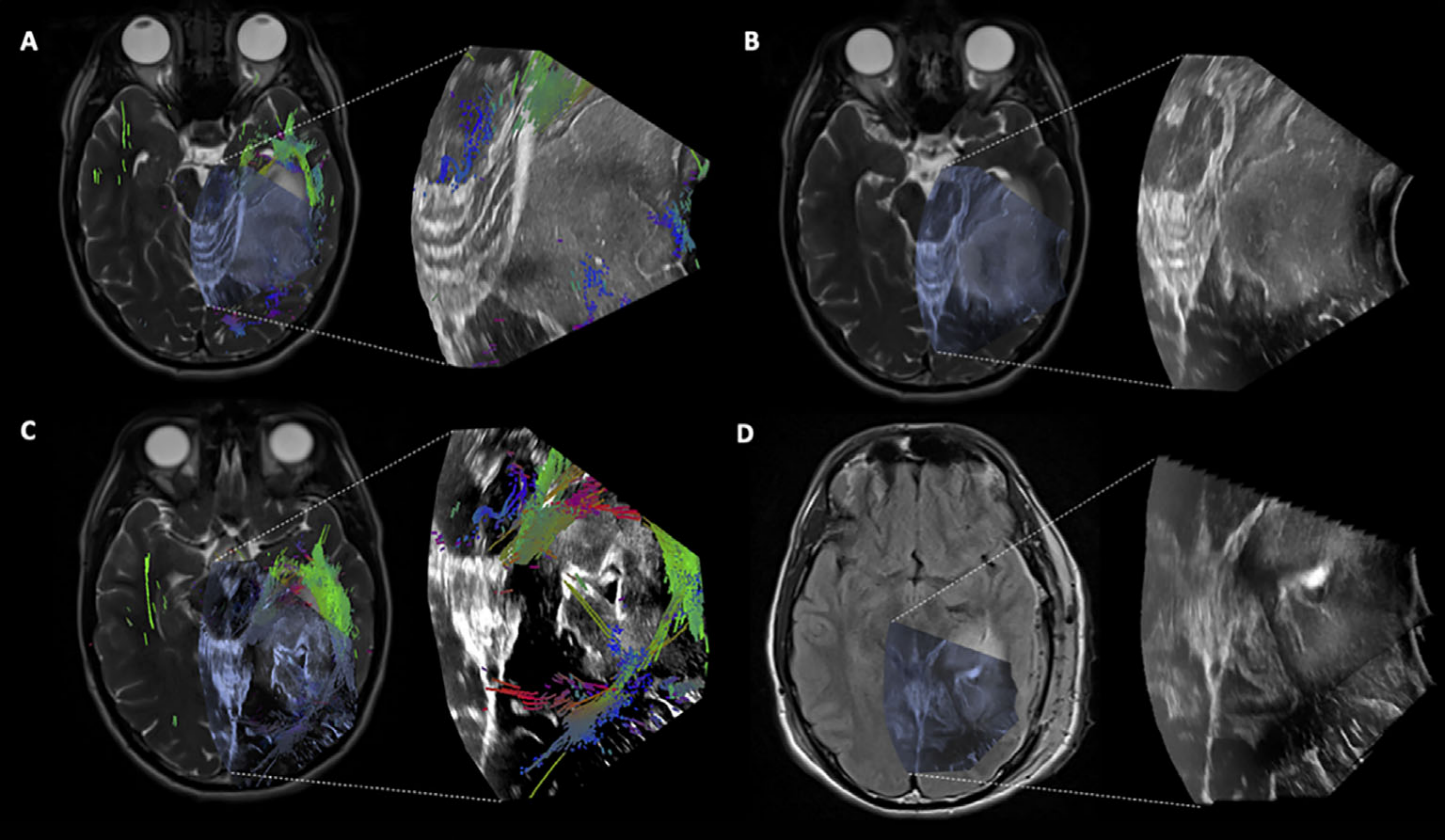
Case 2 Post hoc manually aligned preoperative axial T2-weighted imaging with (A) iUS-1, (B) iUS-2, (C) iUS-3 with multiple tracts around and inside the tumor, and (D) iMRI axial T2-FLAIR imaging manually aligned with iUS-3 without the overlaid tracts. Notice on C the presence of tracts running through the tumor. On D, it is possible to notice the presence of residual tumor in the posterolateral margin as well as posteriorly along the medial margin in the vicinity of the coursing tracts through the tumor.
